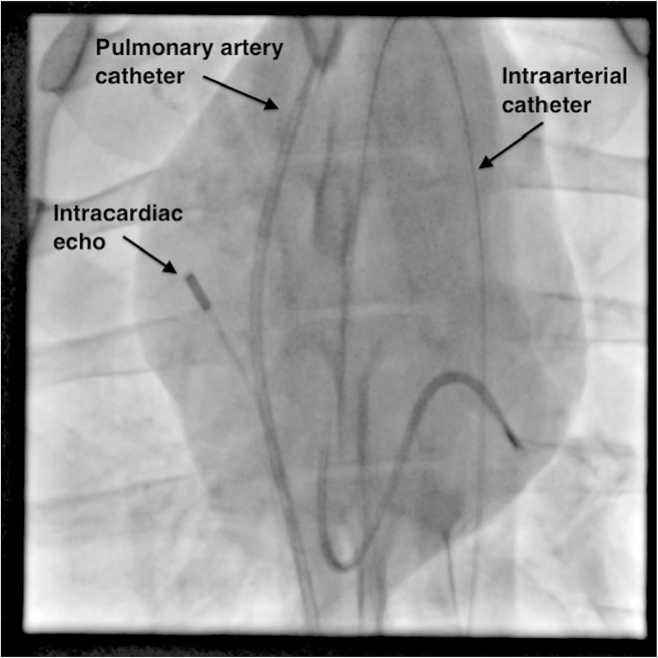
Fluroscopy images of intracardiac echocardiography (ICE), pulmonary artery and arterial catheters used during the study.
